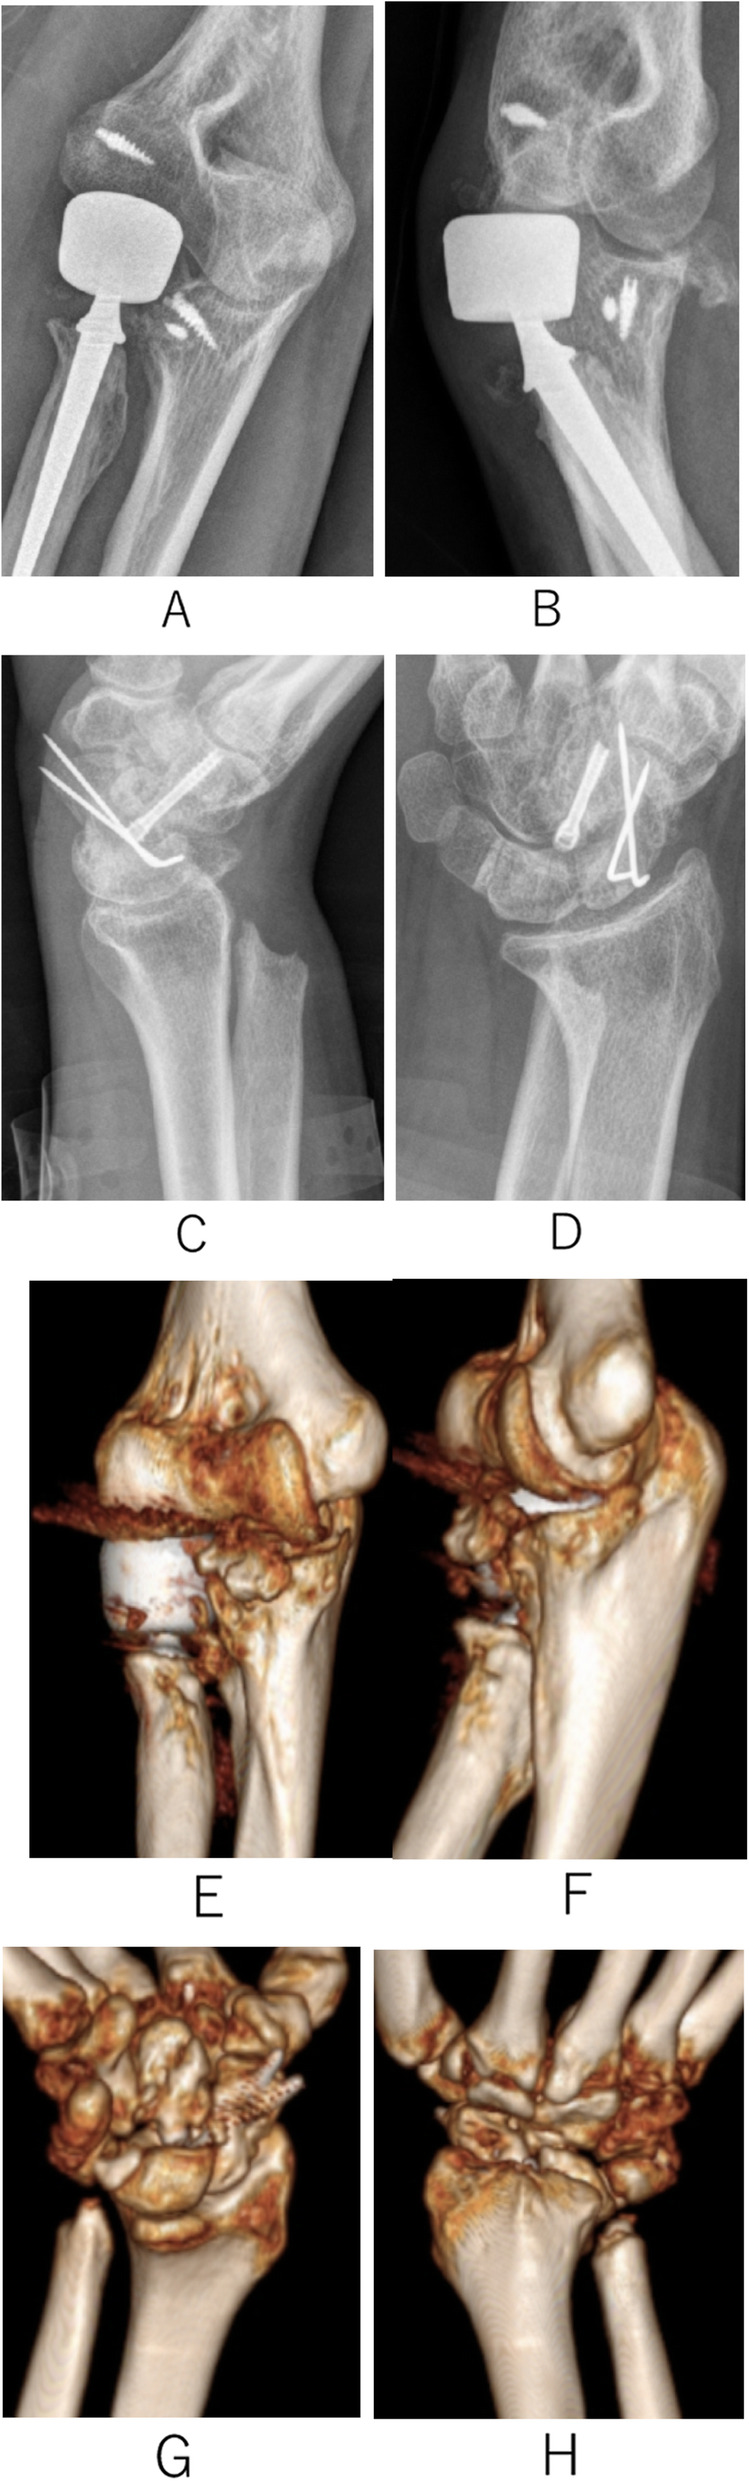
One year after second surgery radiographs. a-b, elbow joint; c-d, Wrist joint; e-f, CT: elbow joint; g-h, CT: wrist joint
